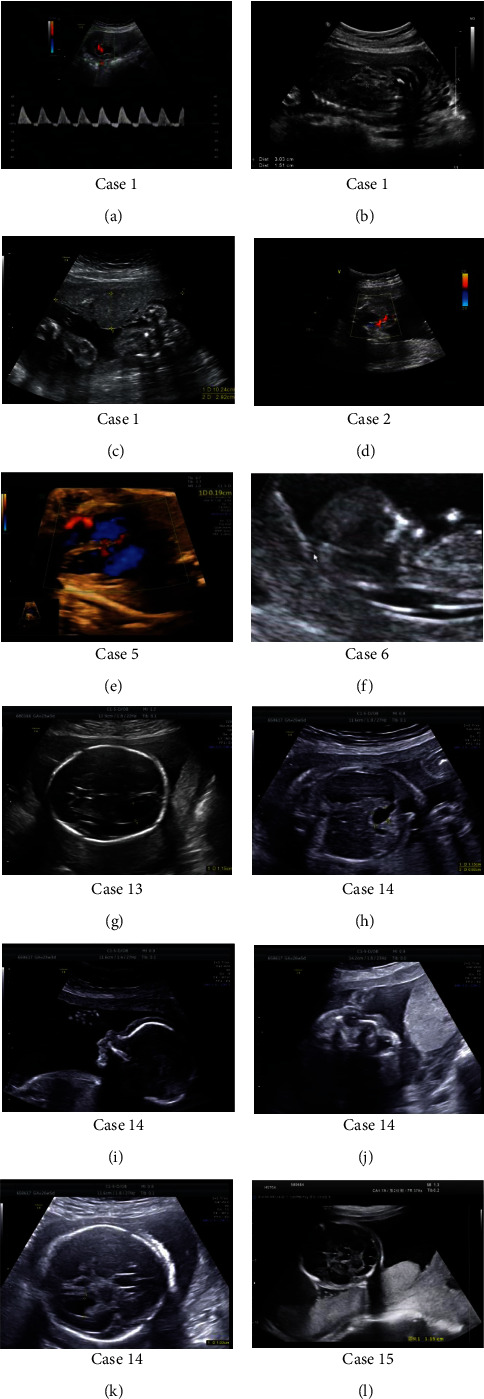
Representative prenatal ultrasonographic indications in these cases. Case 1: (a) umbilical artery blood flow spectrum in diastolic phase disappeared and reversed; (b) increased intestinal echo; (c) small placenta. Case 2: (d) single umbilical artery. Case 5: (e) ventricular septal defect. Case 6: (f) nuchal translucency thickening. Case 13: (g) dilated left lateral ventricle. Case 14: (h) duodenal stricture; (i) micrognathia; (j) wide eye distance; (k) “Strawberry head,” dilated lateral ventricles. Case 15: (l) cerebellar medulla pond widening.
